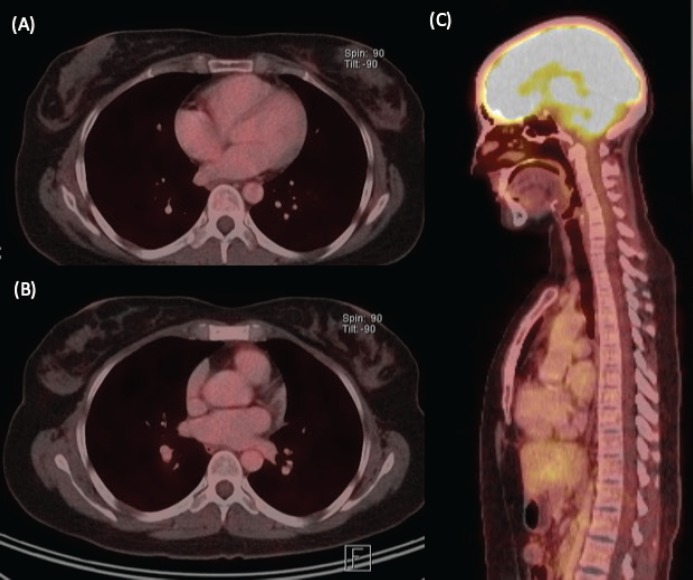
Re-staging imaging study, after 6 months of ST. (A) Axial view of PET/CT with size decrease and hypermetabolism resolution at the left breast nodule. (B) Axial and (C) sagittal views of PET/CT showing hypermetabolism resolution at the sternal body lesion.
